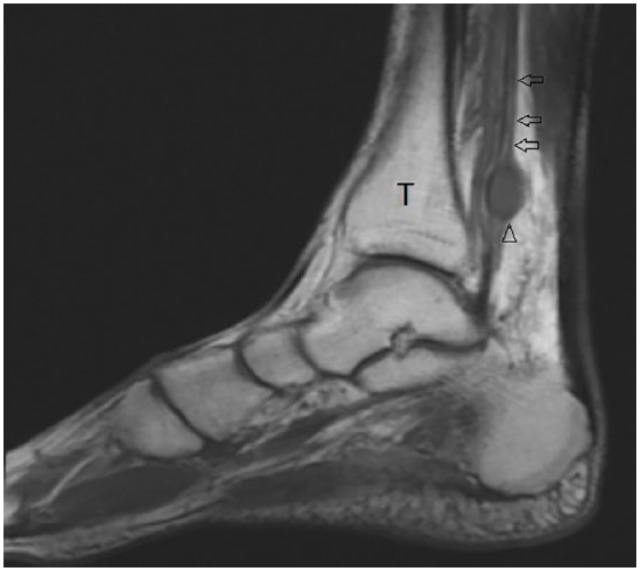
Sagittal T1 MRI image, showing tibial nerve lesion (arrow head) and posterior tibial nerve (arrows) and tibia bone (T).
